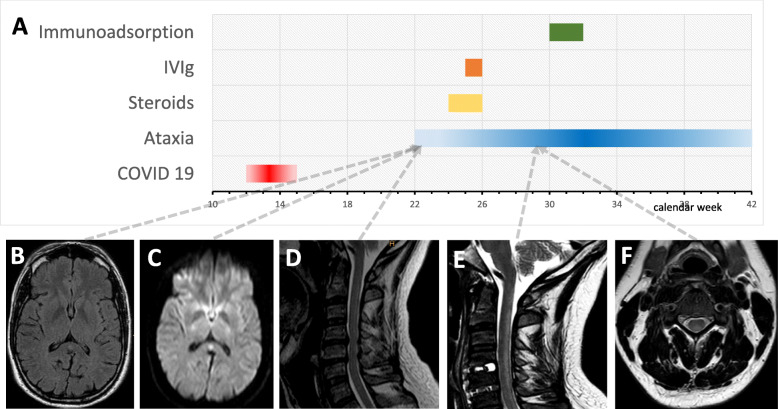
a Disease course and treatment of the patient. b Axial fluid-attenuated inversion recovery (FLAIR) and c axial diffusion-weighted imaging (DWI) show a hyperintensity in the splenium of the corpus callosum. d Sagittal T2 sequence of the cervical spine shows disc protrusion with spinal canal stenosis at C4-C6. e Postoperative sagittal and f axial T2 images of the cervical spine show a new hyperintensity in the dorsal columns extending from the cranial base to C6/7
